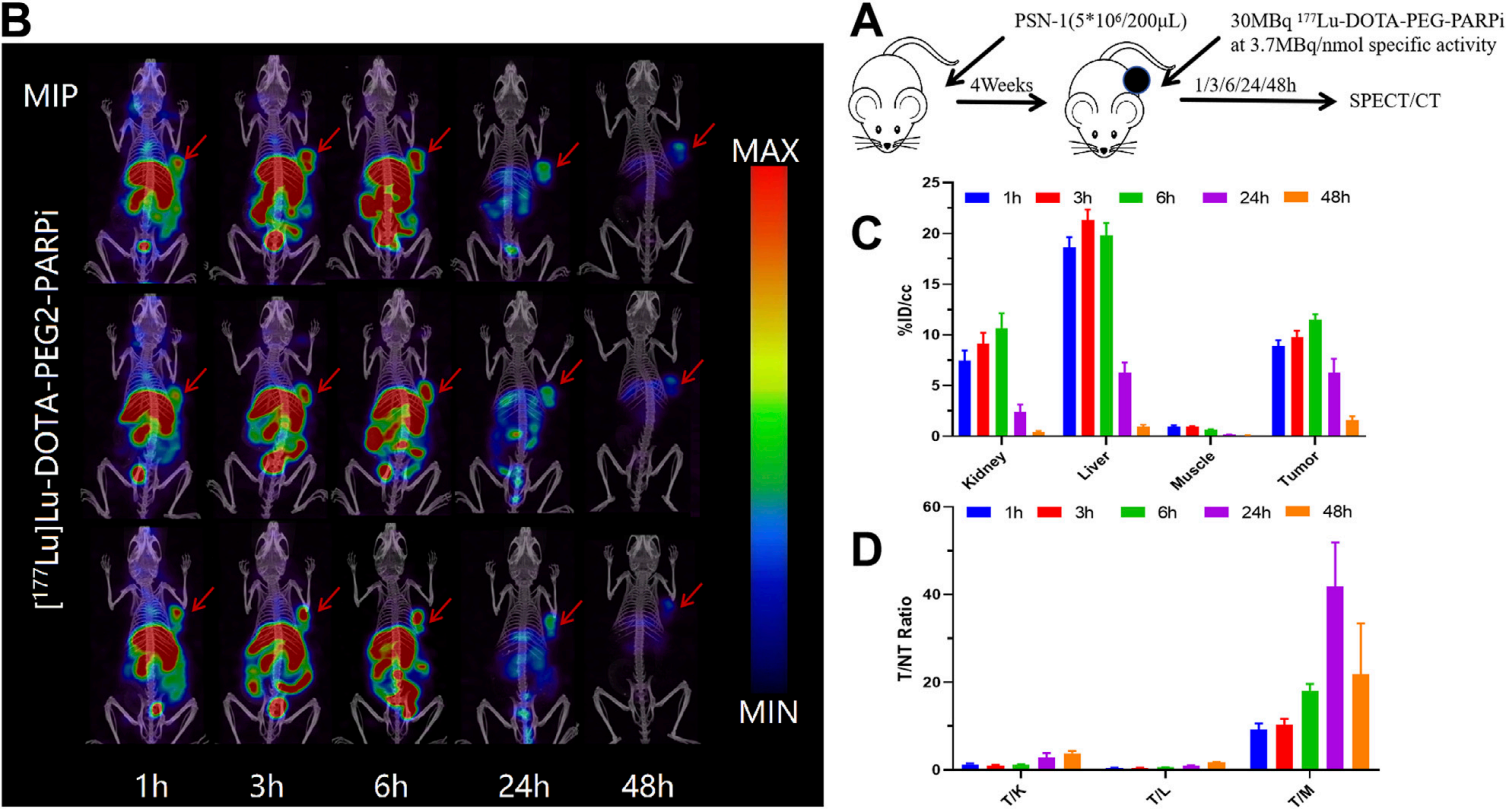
SPECT/CT result of ^177^Lu-DOTA-PEG-PARPi in PSN-1 models. Molecule imaging of ^177^Lu-DOTA-PEG-PARPi (A). Micro SPECT/CT images of ^177^Lu-DOTA-PEG-PARPi in PSN-1 models at 1, 3, 6, 24, and 48 h after administration (B). Ratios of tumor-to-non-target tissue (n = 3). Quantification of the regions of interest (ROIs) in PSN-1 models expressed as %ID/cc (C). T/M (tumor-to-muscle) ratios (D).
